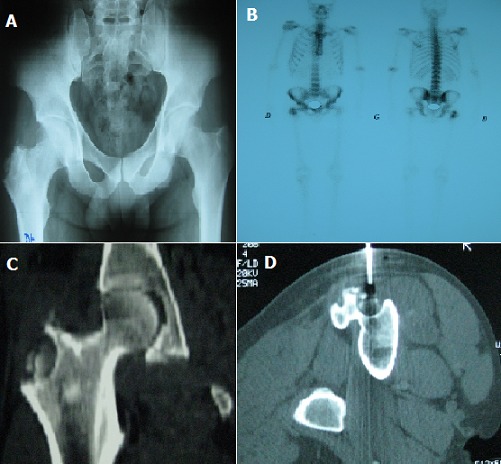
(A) X-ray of the pelvis: osteolytic lesion of the right great trochanter with thickening of the soft parts; (B) bone scintigraphy: hyperfixation of the right great trochanter; (C) scanner of the basin: osteolytic lesion of the right great trochanter containing multiple sequesters with cortical irregularity; (D) a great-trochanter bone biopsy under scannographic control
